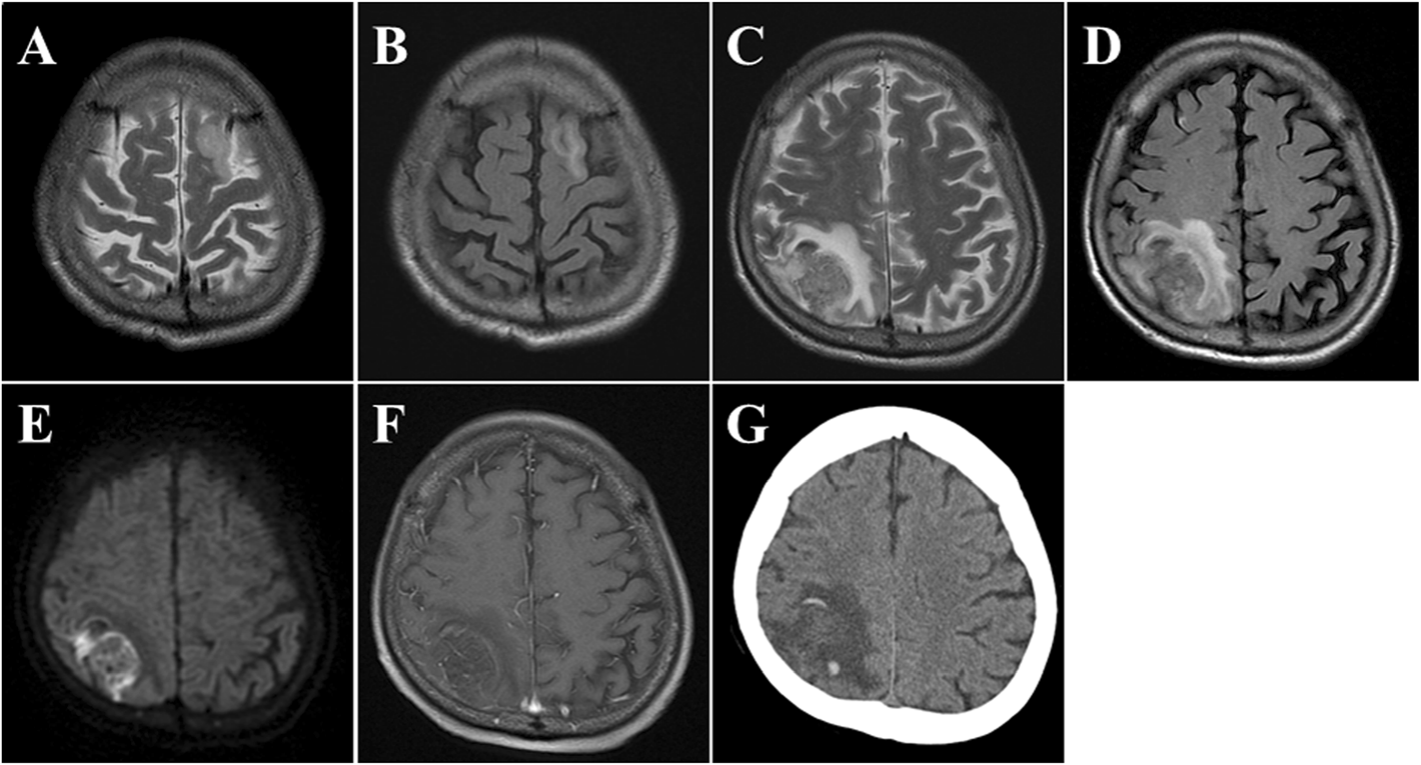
T2 weighted magnetic resonance imaging (MRI) (T2WI) and fluid-attenuated inversion recovery (FLAIR) 3 months before admission revealed a white matter lesion in the left superior frontal gyrus (a, b). T2WI and FLAIR on admission revealed a right parietal and frontal white matter high intensity lesion (c, d). Diffusion MRI showed a high-intensity lesion in the right parietal gray matter (e), and T1-weighted MRI after gadolinium administration revealed no enhancing structures (f). CT revealed high density area in the right parietal and frontal lesion (g)
